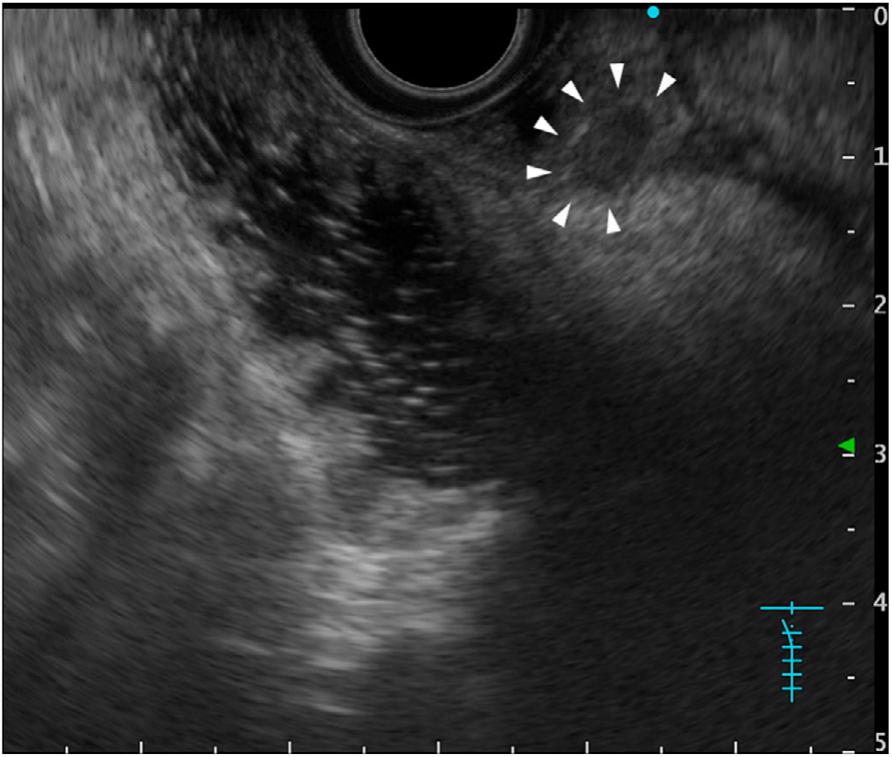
Endoscopic ultrasonography image. A 6.2‐mm hypoechoic tumor is seen within the submucosal layer of the ampulla of Vater (arrowhead). Endoscopic ultrasonography indicated no invasion to the muscularis propria layer of the duodenum and intraductal extension to the bile or pancreatic duct
